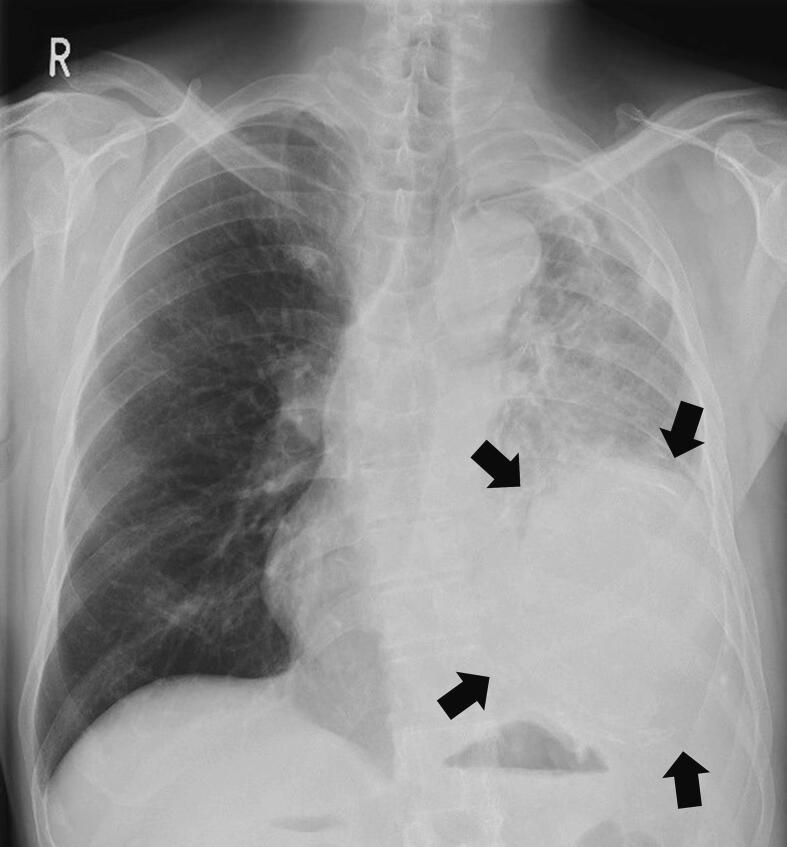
Chest roentgenogram depicting a huge mass shadow (black arrows) in the left lower lung field.
